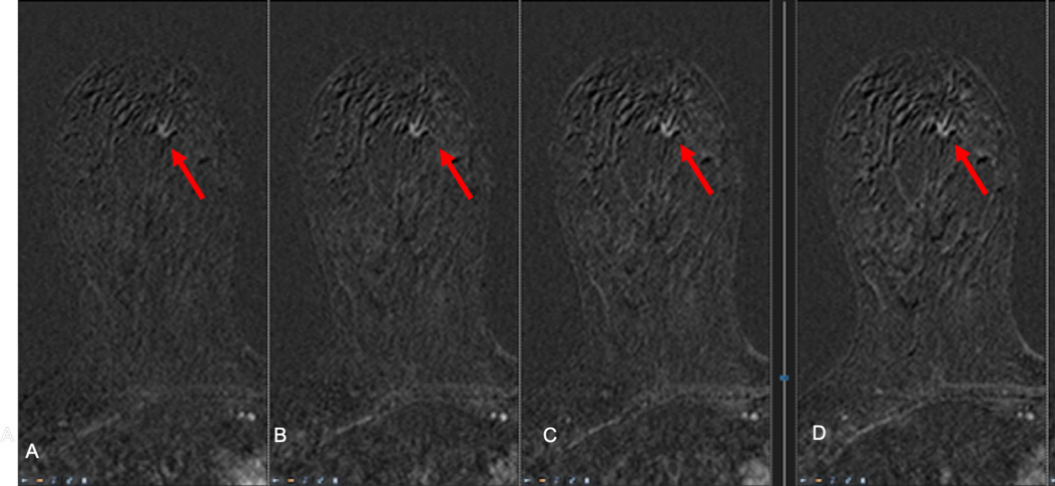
MRI of the right breast after 4 months from the prior examination (Figure 4) and four cycles of docetaxel + trastuzumab and pertuzumab. *T*_1_ weighted fat sat subtracted at 2 min (A), 4 min (B), 6 min (C) and 8 min (D) after administration of contrast media. The small 9 mm non-mass enhancing lesion is now less visible showing a different uptake of contrast media which is now slow and progressive overall the dynamic examination. The is stable in size (5–6 mm).
